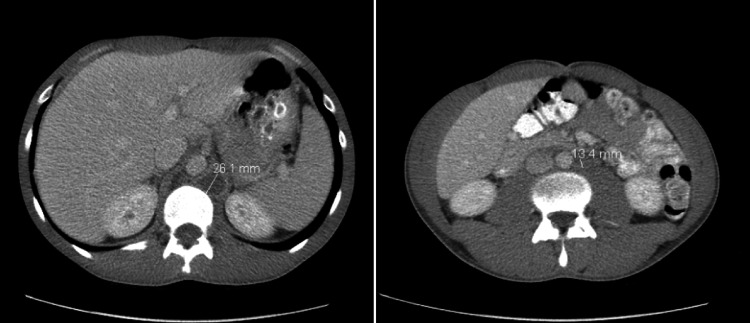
CT of the abdomen and pelvic showing large retroperitoneal lymphadenopathy, the largest conglomeration on the left side measuring 7.8 cm x 6.5 cm, encasing the left ureter causing moderate obstructive hydronephrosis.
